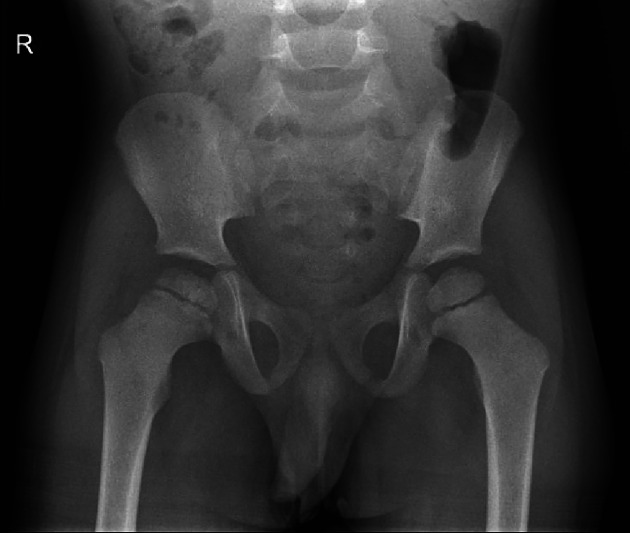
Conventional radiography. Flattening, sclerosis of femoral head; widening of femoral neck.
